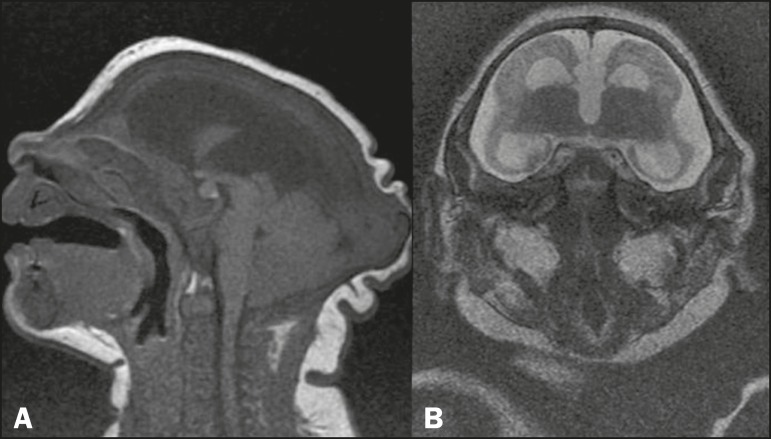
MRI: sagittal T1-weighted sequence (A) and coronal T2-weighted
